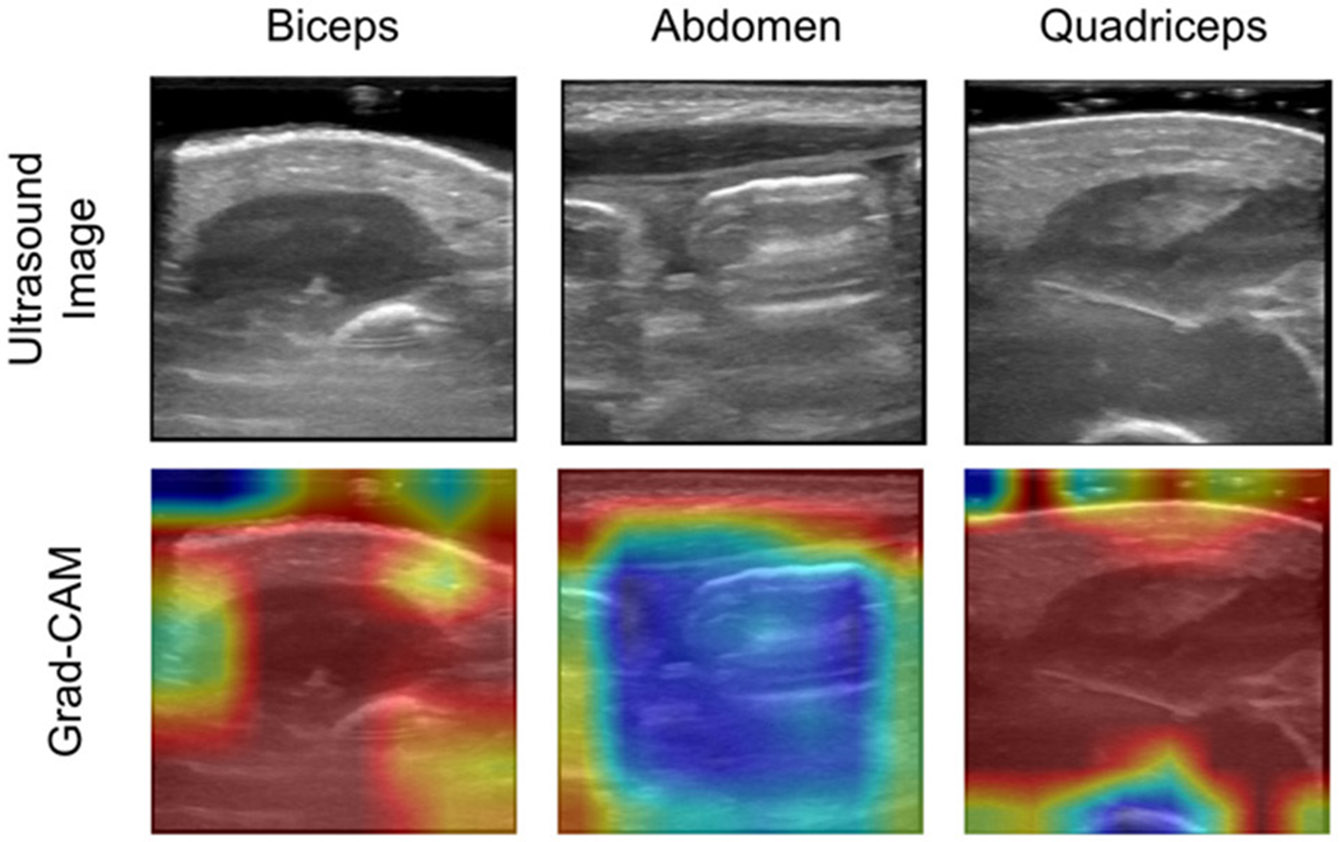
The top row of images shows representative ultrasound images of the biceps, abdomen, and quadriceps. The bottom row shows Grad-CAM results of the contributory regions in the ultrasound images of abdomen, biceps and quadriceps. Warmer colors (reds) indicate higher importance in the model’s prediction, while cooler colors (blue) indicate lower importance in the model’s prediction.
